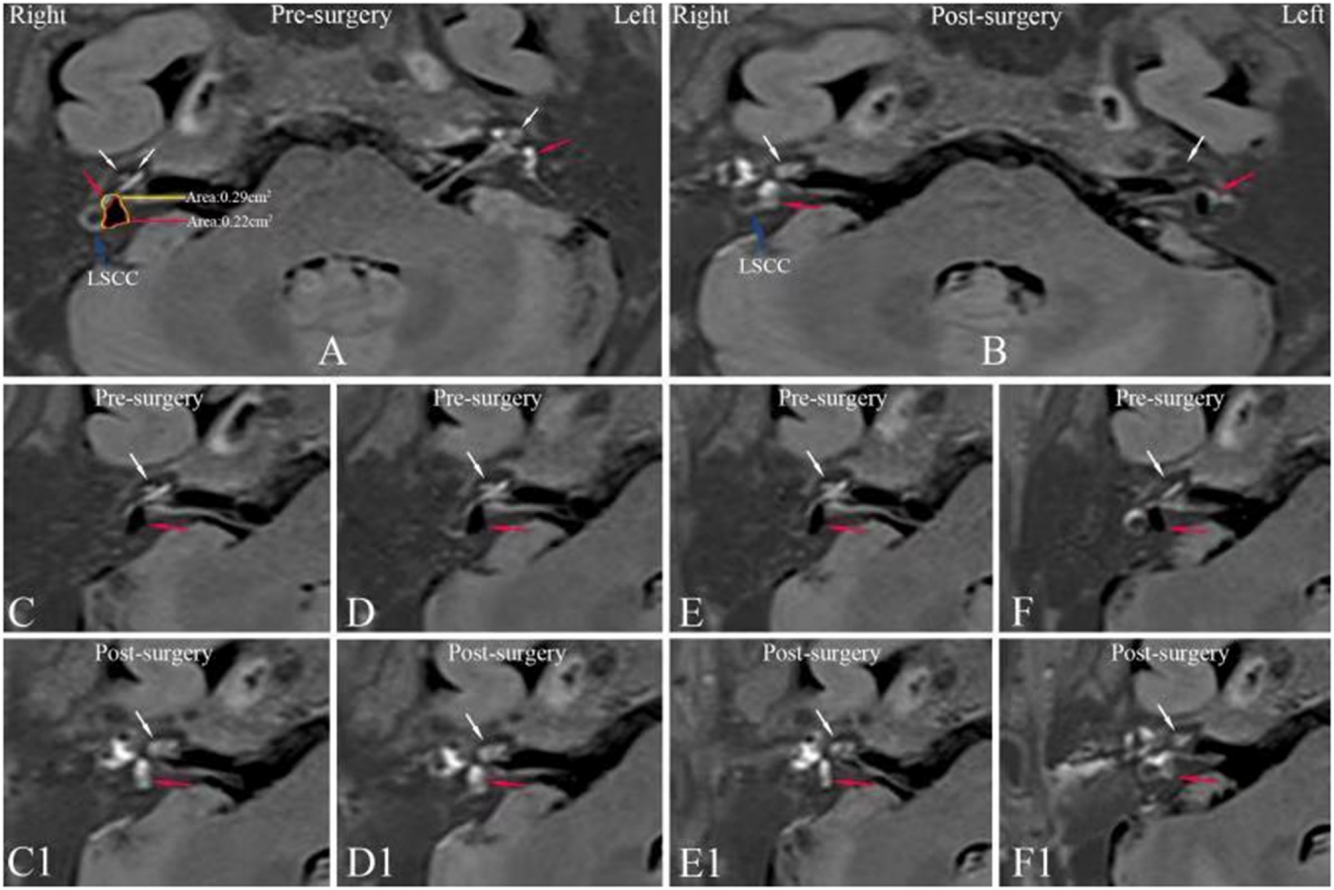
Axial MR images of patient no. 4 (Table 1) with right MD prior to surgery (A,C–F) and 20 months after surgery (B,C1–F1). (A) 3D-real IR MRI revealing a mild cochlear EH (white arrow) and a significant vestibular EH (red arrow) where the vestibular hydrops ratio is 76% in the right ear and no pathological finding in the left ear. (B) In the same slice level as in (A), an axial IT-Gd+IV-Gd MR image of the right affected ear shows bright enhanced perilymphatic fluid in the vestibule (red arrow) and cochlea (white arrow), instead of black non-enhanced area shown in (A), whereas an axial IV-Gd MR image of the left unaffected ear shows insufficient contrast in the inner ear compared with IT-Gd+IV-Gd MR image in (A). Preoperative serial MRIs (C–F) show a mild cochlear EH (white arrow) and a significant vestibular EH (red arrow) in right affected ear. Postoperative serial MRIs (C1–F1) show a disappearance of EH in the vestibule (red arrow) and cochlea (white arrow). LSCC, lateral semicircular canal; 3D-real IR, three-dimensional real inversion recovery; MRI, magnetic resonance imaging; MD, Meniere's disease; EH, endolymphatic hydrops; IT-Gd, intratympanic injection of gadopentetate dimeglumine; IV-Gd, intravenous injection of gadopentetate dimeglumine.
